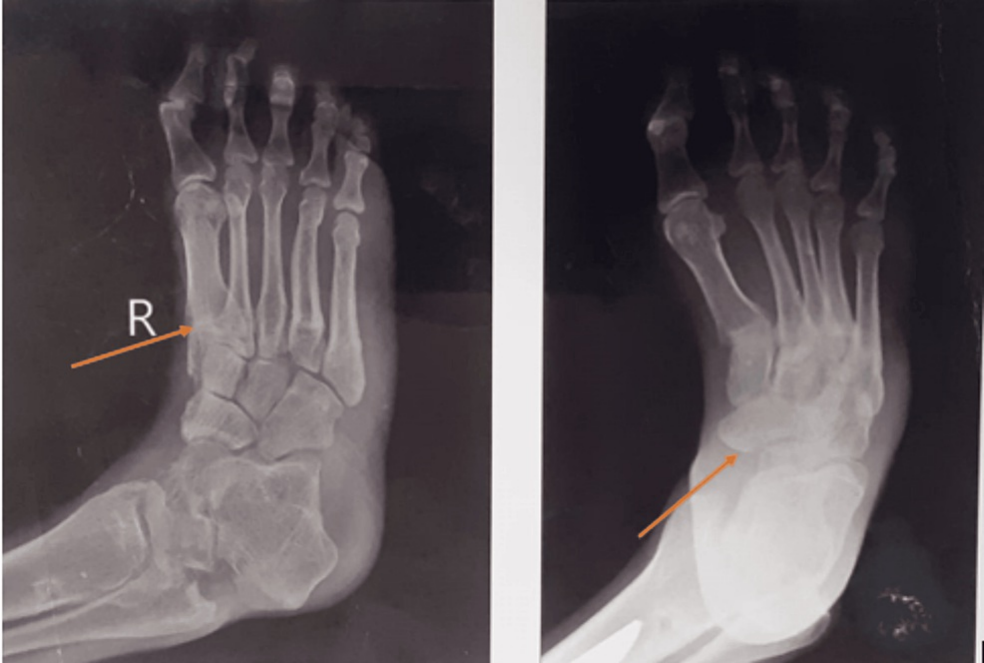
Pre-operative X-ray of Charcot's foot with equinovarus deformity.
